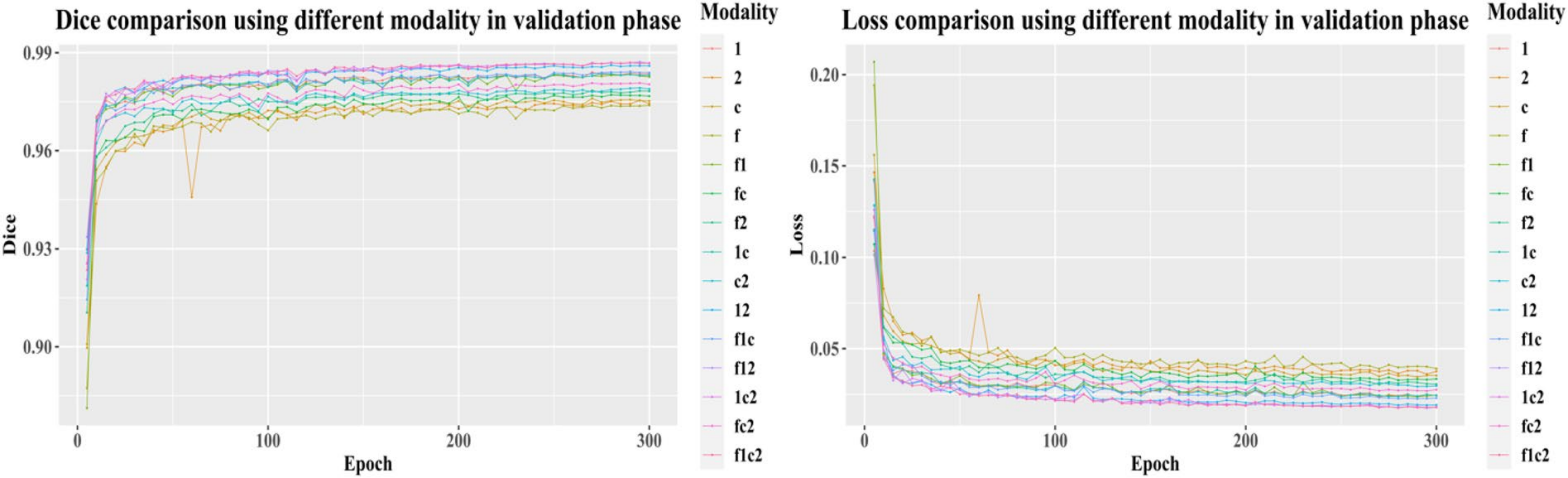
The change of dice (left) and loss (right) in the validation phase. To save training time, we execute the validation part in every 5 epochs. In the legend, 1, 2, c, and f represent T1, T2, T1ce, and T2-FLAIR, respectively. For example, f1ce means that the combination has all four image sequences.
